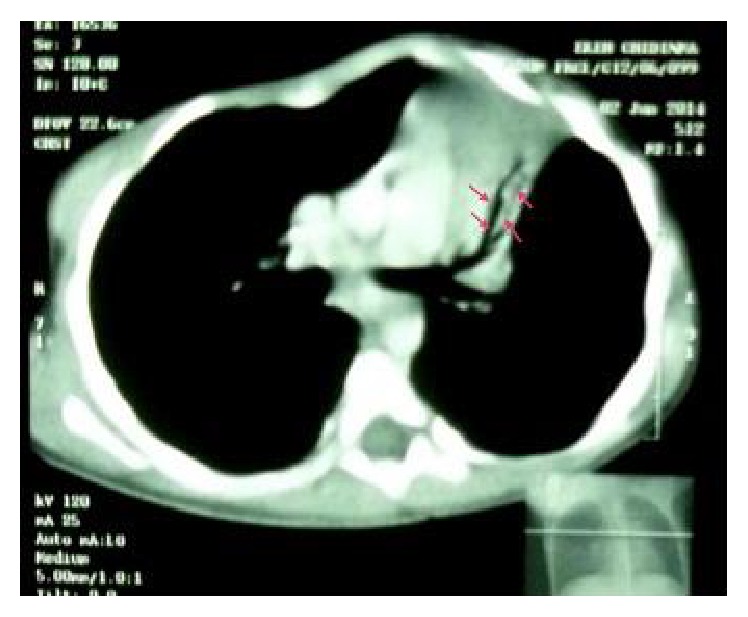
Chest CT scan showing collapse consolidation of upper left lobe with prominent air bronchograms (arrow). Note compensatory hyperinflation of the right upper lobe with shift of the mediastinum to the ipsilateral side.
